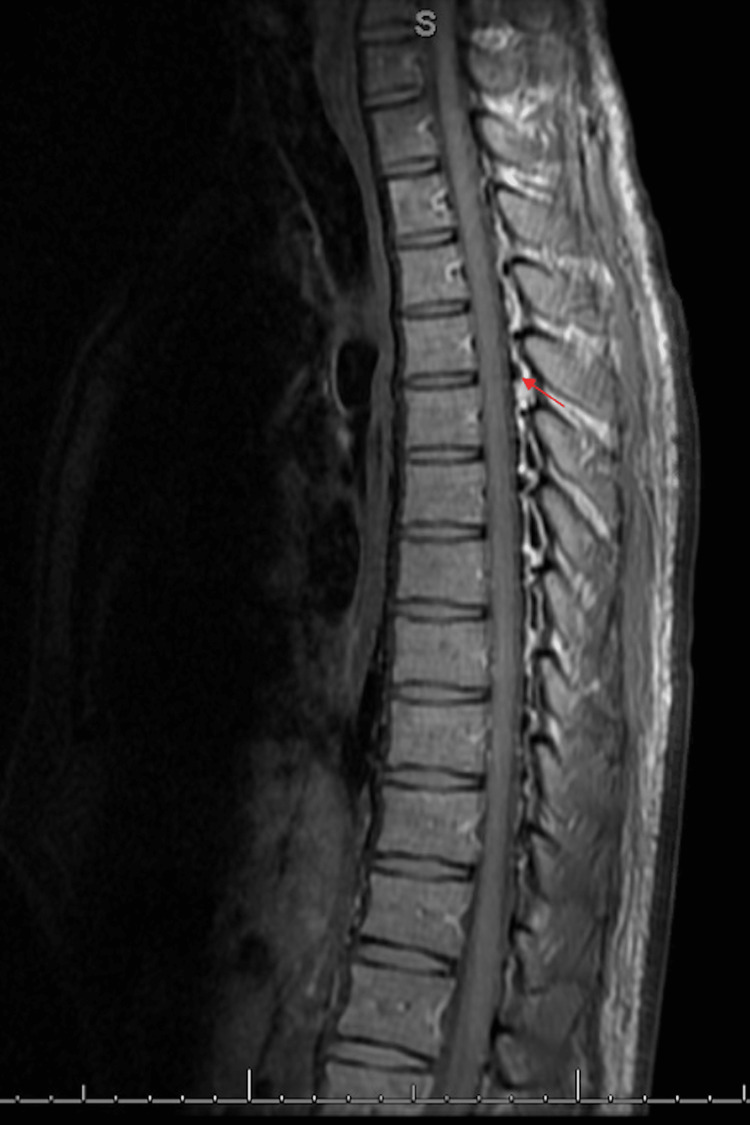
Sagittal magnetic resonance imaging of the spine demonstrating pneumorrhachis (red arrow)
